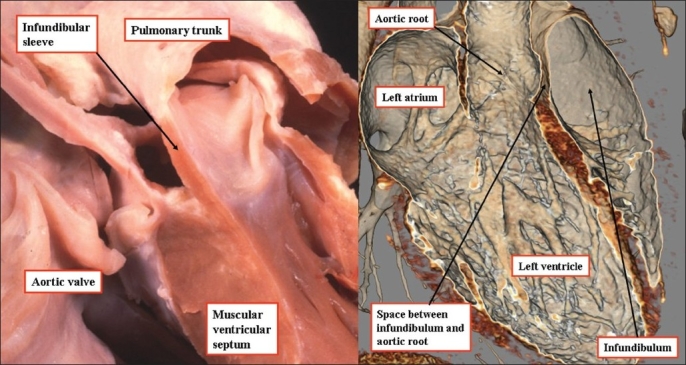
The dissection of the heart seen to the left hand was made by removing the ventricular septum interposed between the ventricular outflow tracts. It reveals how a sleeve of free-standing infundibular musculature lifts the leaflets of the pulmonary valve away from the base of the left ventricle. The computed tomogram, shown to the right hand, and performed to exclude a coronary arterial malformation, replicates the anatomic dissection
